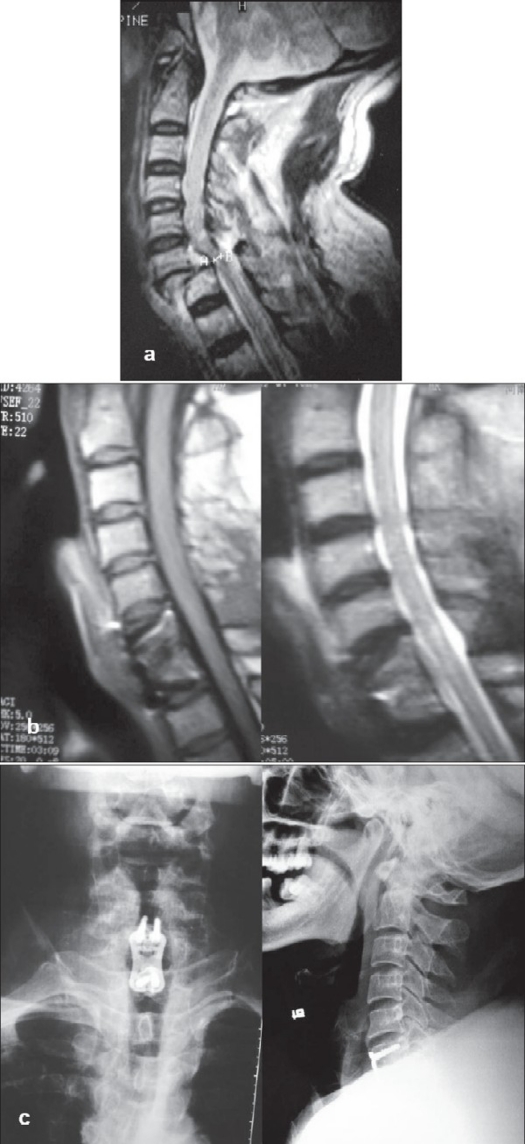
(a) T2WI of mid saggital MRI of cervical spine shows C_6-7_ fractures dislocation with cord compression. (b) T1WI and T2WI mid saggital MRI of the same patients shows reduction and adequate decompression of the cord. (c) X-ray cervical spine AP and Lateral of the same patient showing fusion and stabilization with plate after anterior decompression
